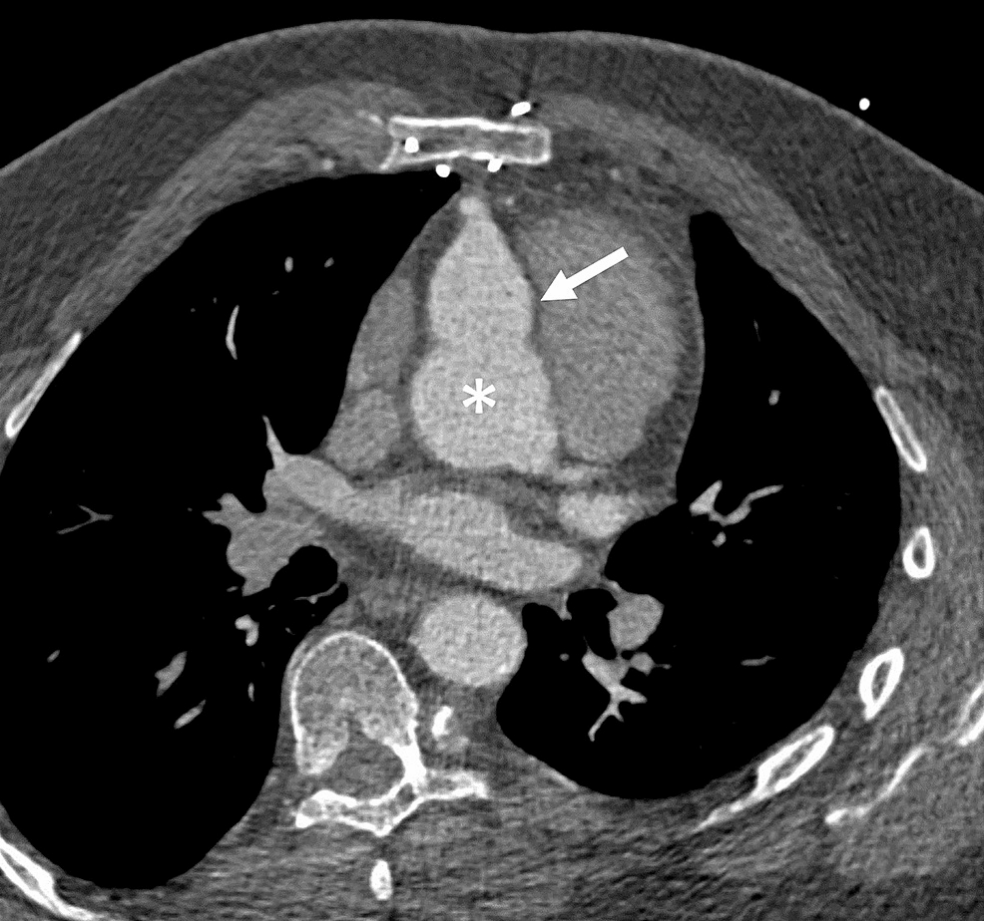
Multiplanar Reconstruction Axial CTA Image Ostial dilation and proximal aneurysm of the right coronary artery (white arrow) approaching the diameter of the proximal aorta (asterisk).
